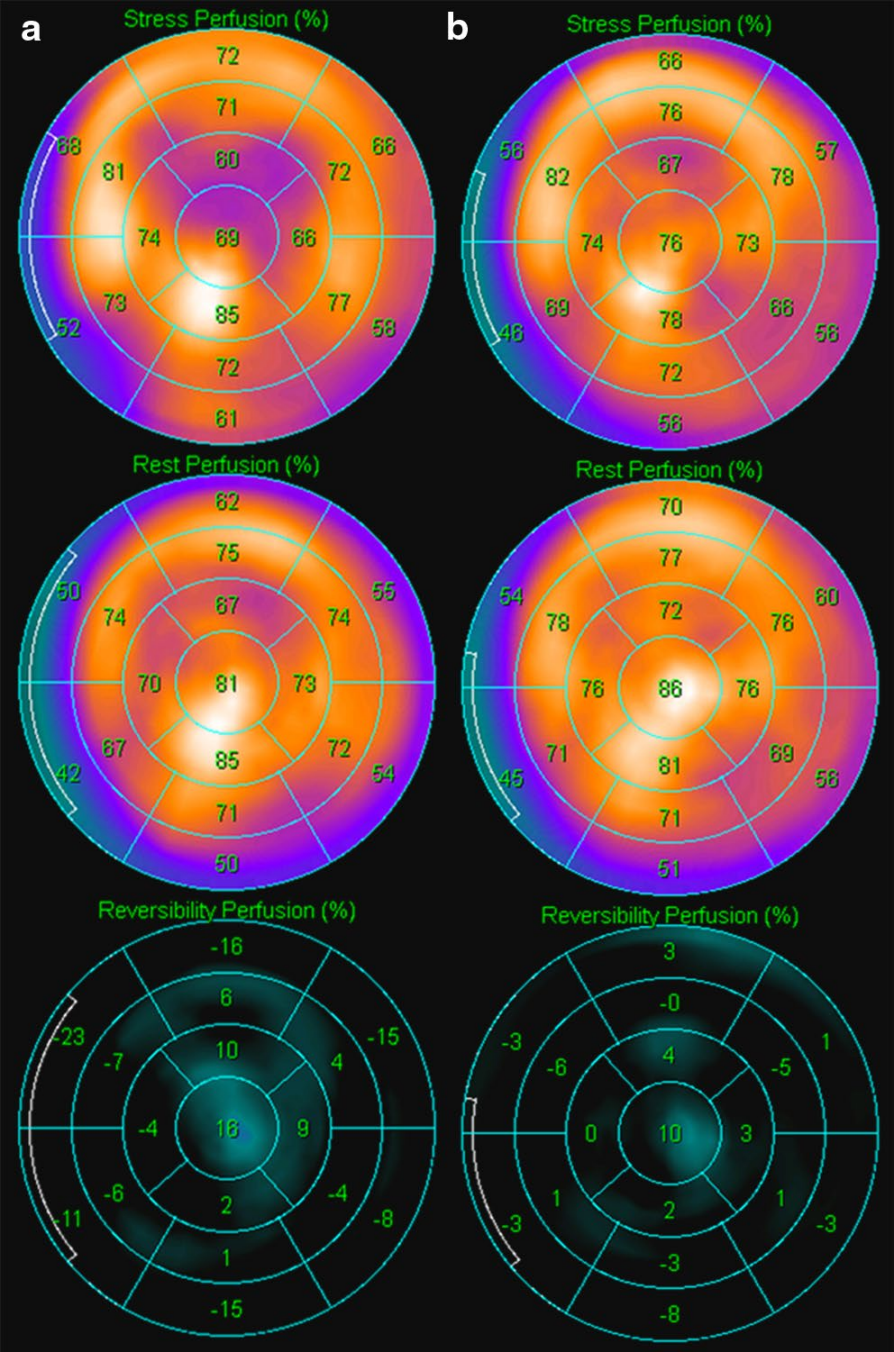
Quantitative polar map (17 segments) displaying the myocardial perfusion as the raw counts based on myocardial scintigraphy analysis. The pixel with the maximum raw count is set to the maximum colour scale brightness and corresponds to a value of 100. The numbers correspond with the average ‘raw’ pixel value in the related segments
